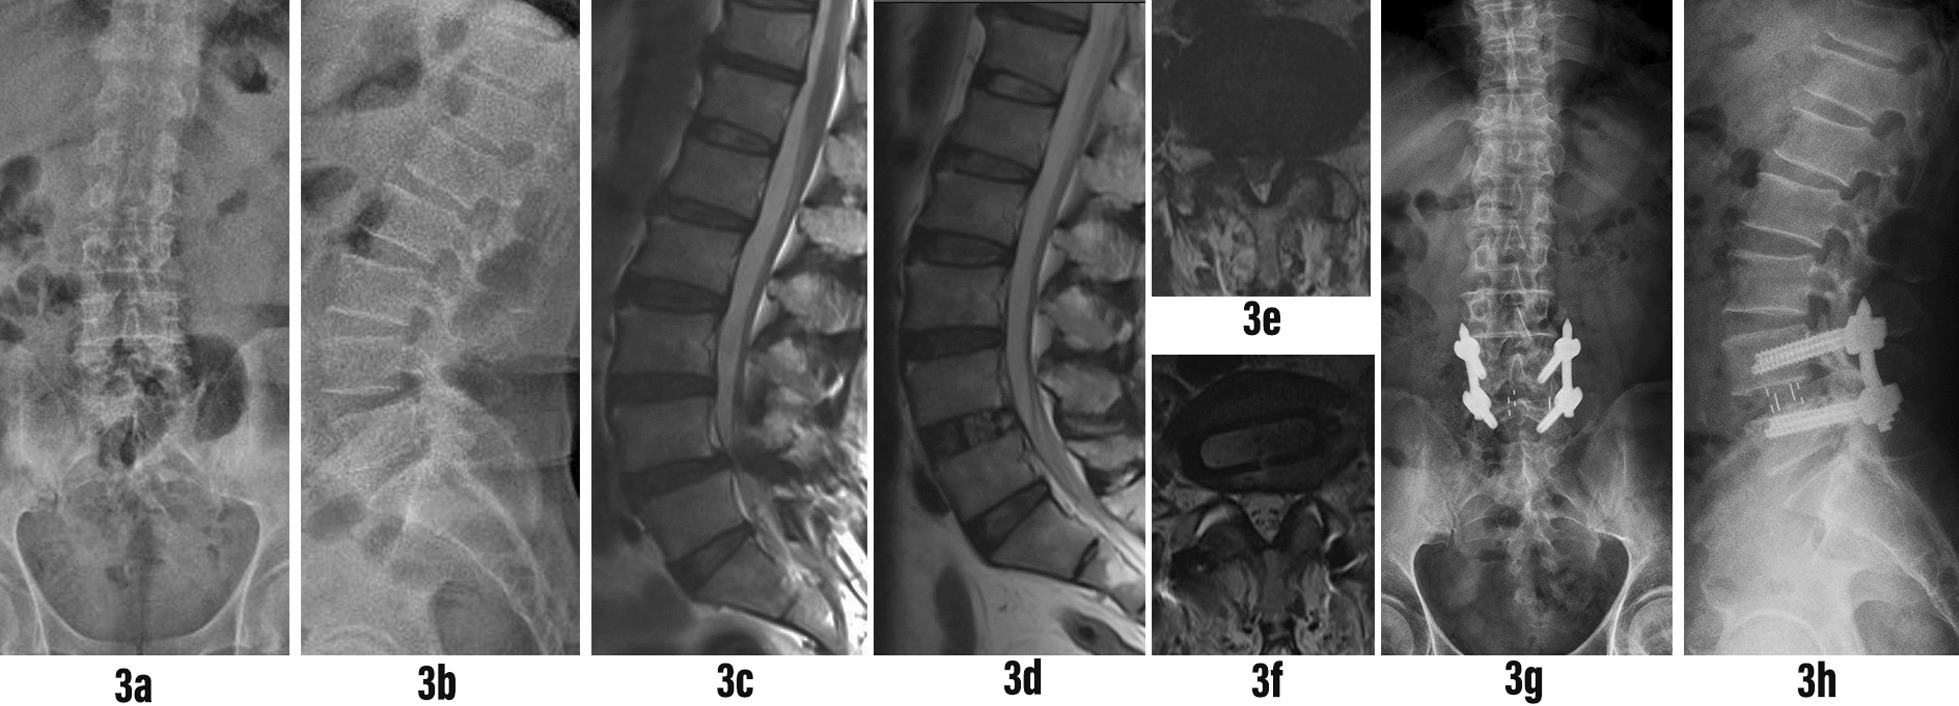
Typical case 3: A 74-year-old male presented with numbness in both lower limbs for more than 1 year, aggravated for 1 week. Diagnosis of L4-5 spinal stenosis with L4 vertebral 1 degree spondylolisthesis. L4-5 single segment OLIF combined with posterior L4-5 percutaneous internal fixation was performed. 3a, 3b Anteroposterior and lateral X-ray films of the lumbar spine before operation showed lumbar degeneration and I ° spondylolisthesis of L4 vertebral body. 3c Preoperative sagittal T2 MRI of the lumbar spine showed L4-5 spinal stenosis, no RNRs above the stenosis plane. 3d Postoperative sagittal T2 MRI of the lumbar spine showed no RNRs above the L4-5 surgical segment. 3e, 3f Axial T2 MRI of lumbar spine before and after operation showed that the dural sac CSA increased significantly after OLIF indirect decompression. 3g, 3h At the last follow-up, the X-ray film of the lumbar spine showed that the L4-5 pedicle screw was fixed, good reduction of L4-5 spondylolisthesis, the fusion cage was placed in a good position, and the height of the L4-5 intervertebral space was well restored
